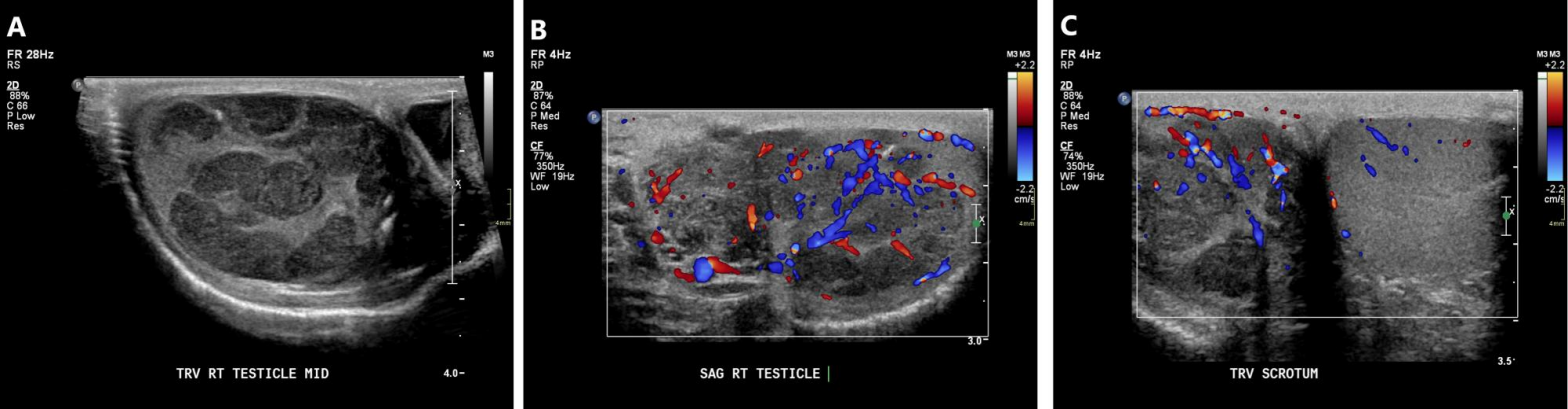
Right testicular seminoma. (A) There are multiple defined hypoechoic lesions within the right testis. (B) Doppler interrogation demonstrates vascularity indicating that these are not cystic lesions. (C) Concurrent comparison with the normal left testis accentuates the aforementioned findings.
